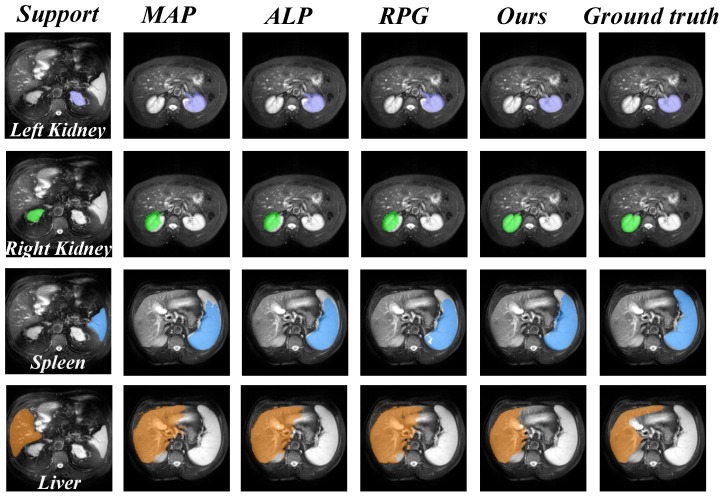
Comparison of validity of prototype extraction methods between our method and other methods on the CHAOS-MRI dataset.
